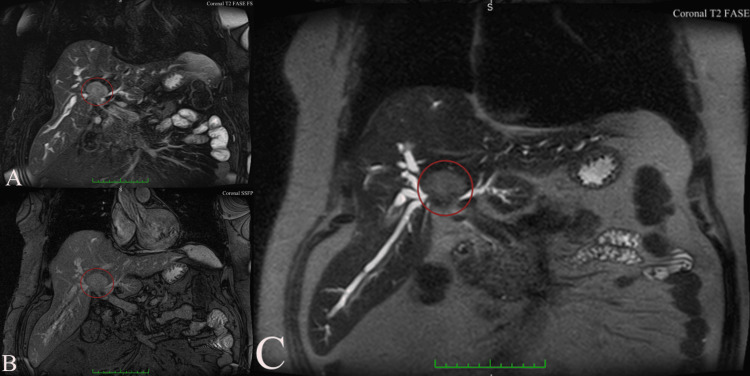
Coronal MR imaging sequences from the same patient with Bismuth-Corlette type IIIa perihilar cholangiocarcinoma. (A) T2-weighted fat-suppressed coronal image demonstrating marked bilateral intrahepatic biliary dilatation with abrupt cutoff at the hepatic hilum (red circle). (B) Coronal steady-state free precession (SSFP/TrueFISP) sequence providing an overview of the hilar stricture with complete separation of the right and left hepatic ducts (red circle). (C) Thick-slab heavily T2-weighted coronal MRCP image showing high-grade stricture of the biliary confluence with symmetric upstream dilatation of the right and left ductal systems, classic appearance of a Bismuth-Corlette type IIIa Klatskin tumor (red circle)[3]. Tumor classification is based on the Bismuth–Corlette classification system as originally described by Bismuth et al. (1992) [3]. TrueFISP: True Fast Imaging with Steady state Precession (Siemens Healthineers, Erlangen, Germany); MRCP: Magnetic resonance cholangiopancreatography
